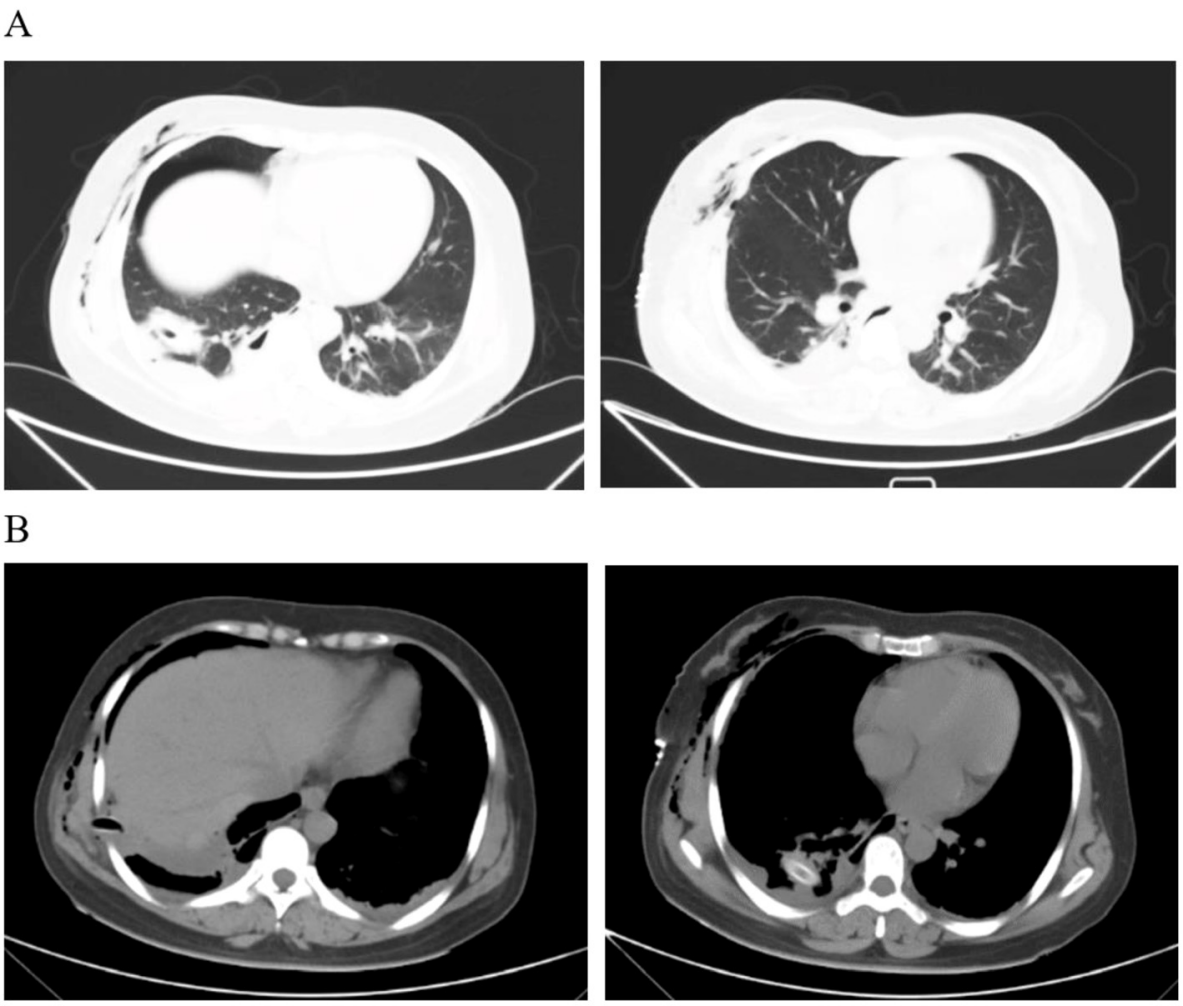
Pleural effusion was scanned by CT on the third day after the operation. (A) The lung window shows a little consolidation of the lungs. (B) The drainage tube can be seen in the mediastinal window, and a small amount of effusion can also be seen.
